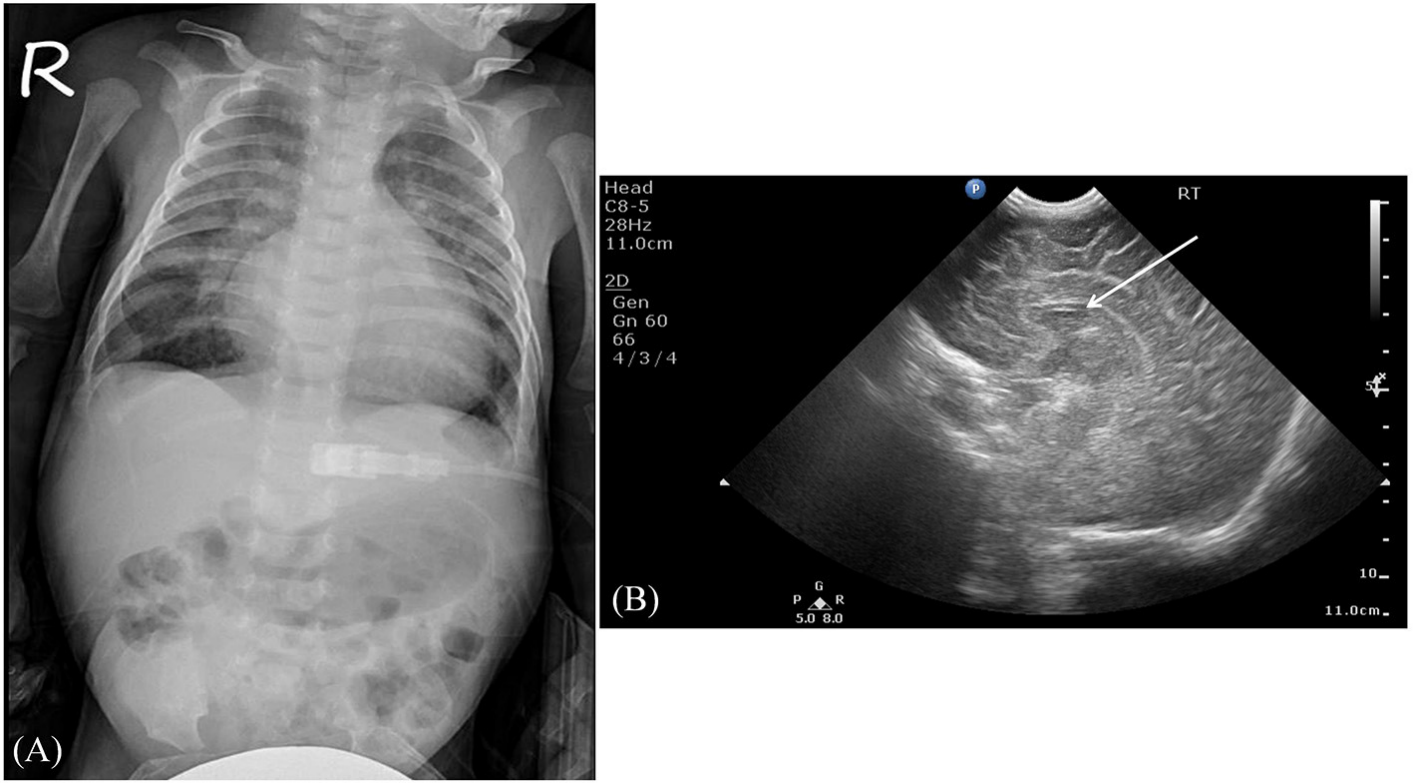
Imaging studies. (A) The chest radiography revealed cardiomegaly and pulmonary congestion. (B) A sagittal view using brain sonography showed a subtle hypoplasia of the corpus callosum (indicated by the white arrow).
